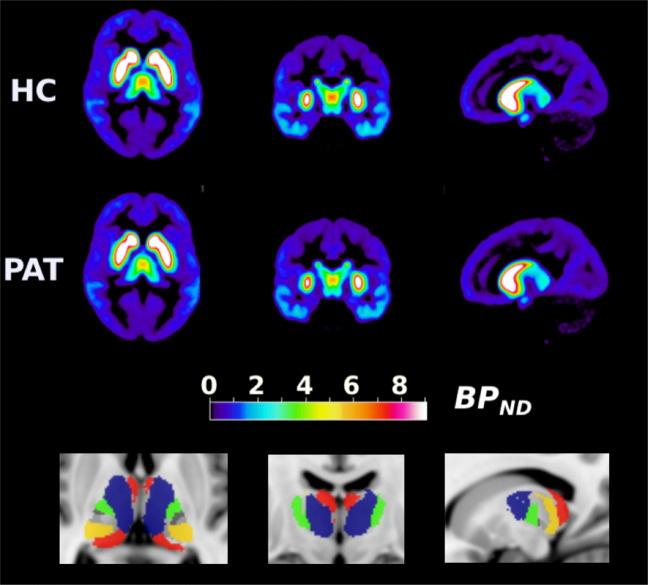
Average BP_ND_ values and thalamic ROIs. Upper panel: average BP_ND_ image values for first-episode psychosis patients and matched control subjects. Lower panel: ROIs for thalamic subregions shown on a template T1 MR image, based on their connectivity to cortical brain regions. Blue = THA-PFC (projections to prefrontal cortex), red = THA-TC (temporal cortex), gray = THA-M1 (primary motor cortex), green = THA-PreMC (premotor cortex), orange = THA-PPC (posterior parietal cortex).
